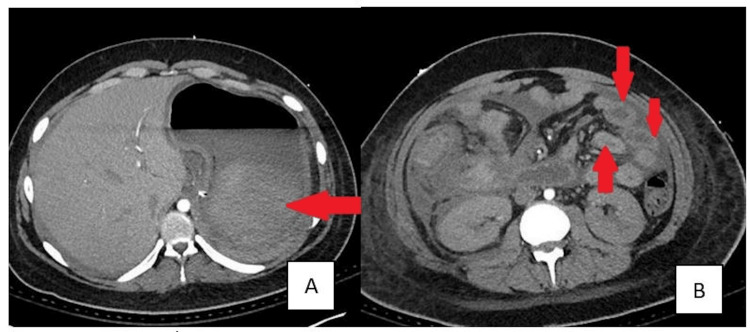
CT abdomen depicting the worsening of mixed-density debris in the gastric lumen [A] and multifocal abscesses [B]. CT, computed tomography.
